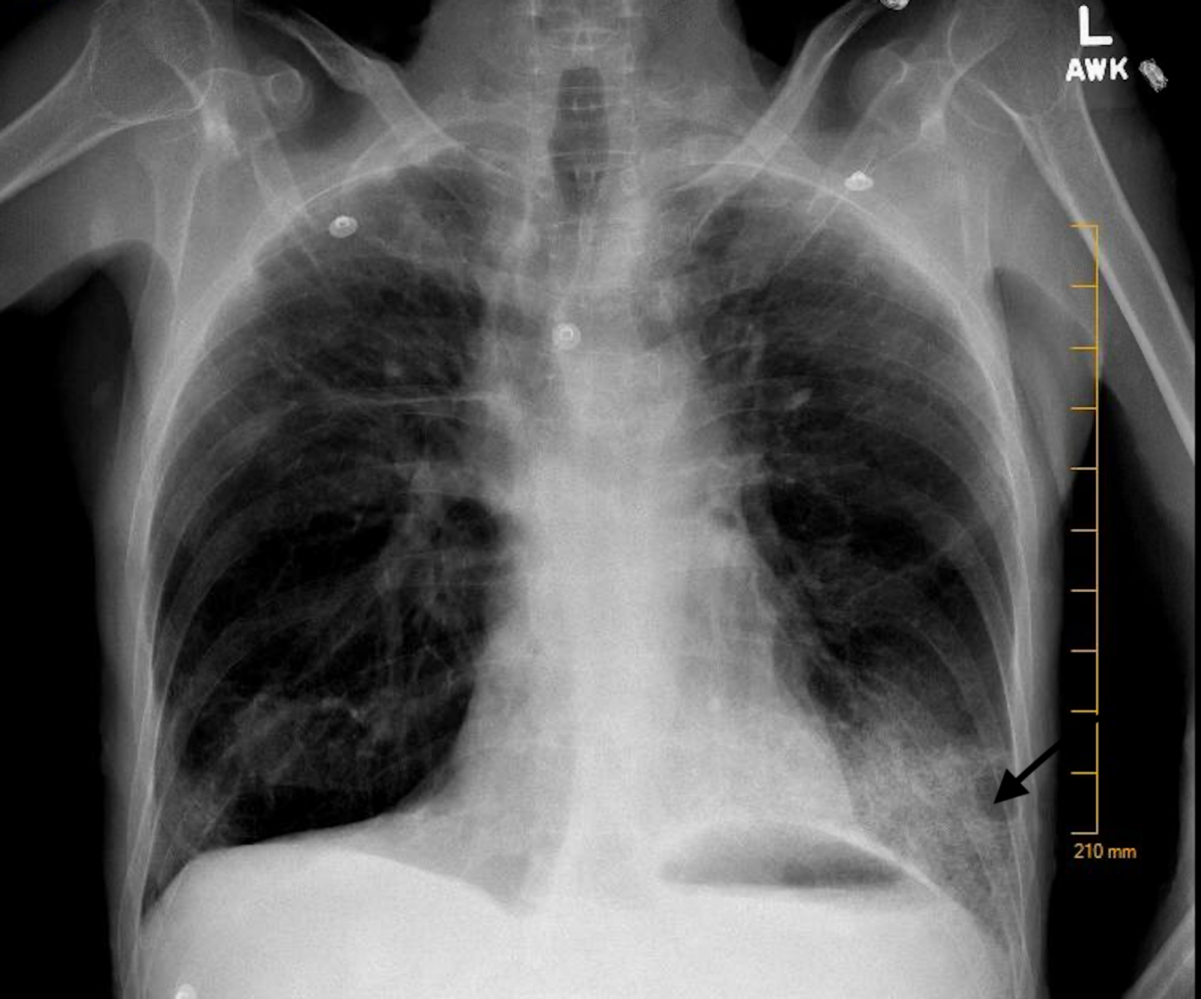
Chest X-ray showing left lower infiltrate.
